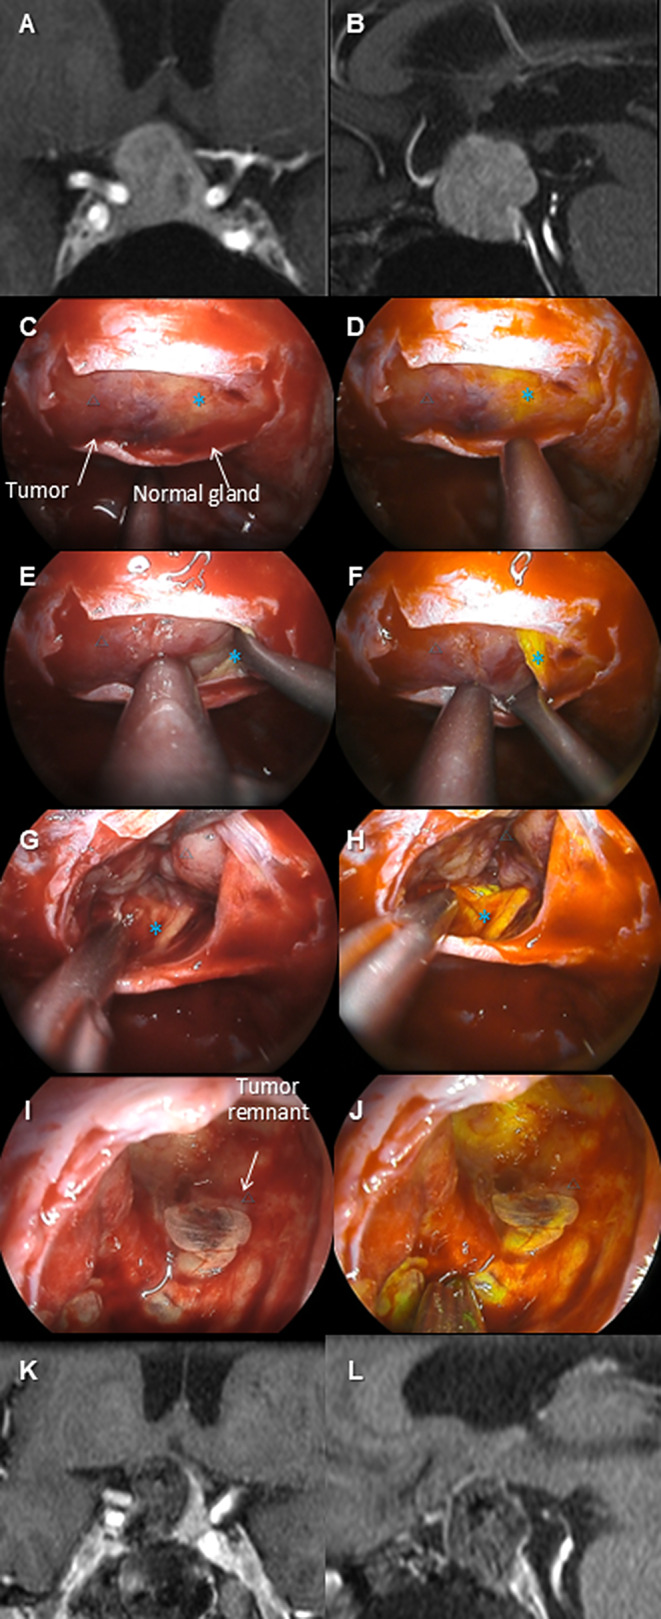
The preoperative MRIs (A and B, gadolinium-enhanced T1-weighted image), intraoperative pictures (C, E, G, and I, normal light; D, F, H, and J, Yellow Enhance [YE] mode), and postoperative MRIs (I and J, gadolinium-enhanced T1-weighted image) of case #2 are shown. This patient was a 73-year-old woman who presented with visual field disturbances accompanied by visual impairment due to pituitary neuroendocrine tumor. Preoperative MRI suggested that the normal pituitary gland was displaced to the left (A, B). During surgery, after wide exposure of the sellar region and dural incision, the tumor was exposed. Under YE mode observation, the yellow-tinted normal pituitary gland (*) was emphasized, enhancing the color contrast with the tumor (△), which facilitated easy differentiation (C–H). The tumor-pituitary boundary was dissected from the anterior side. The capsule became fragile toward the superior part, necessitating some intramembranous resection. The YE mode more clearly visualized a small tumor remnant than the normal light (I, J), and complete resection was ultimately achieved. The visual field disturbance improved postoperatively. The YE mode distinctly enhances the color contrast between the tumor and the normal gland
